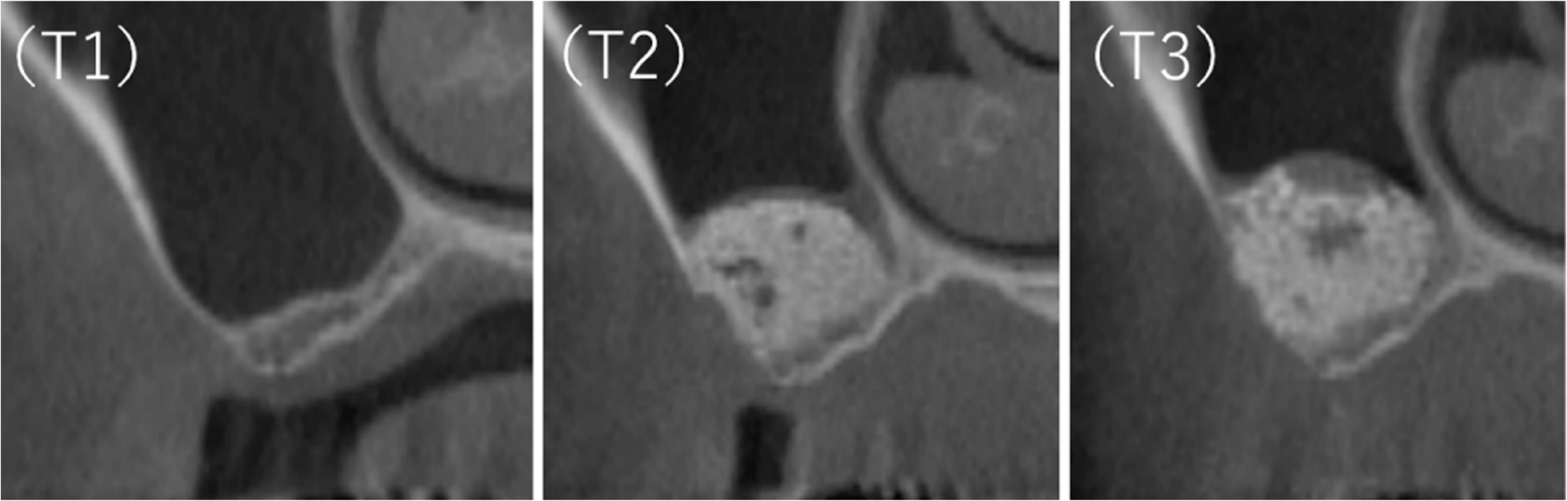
CBCT images of the maxillary sinus: (T1) before surgery, (T2) immediately after surgery, and (T3) 6 months after surgery
